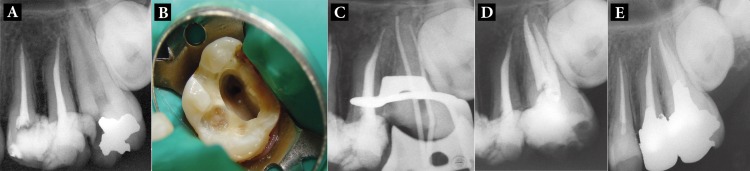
A) Radiographic images of First maxillary molar with two-canal; B) Two orifices were showed in the access cavity image; C) The master cones were inserted at the one buccal and one palatal canal; D) Post-operative radiograph after treatment; E) One-year follow-up
